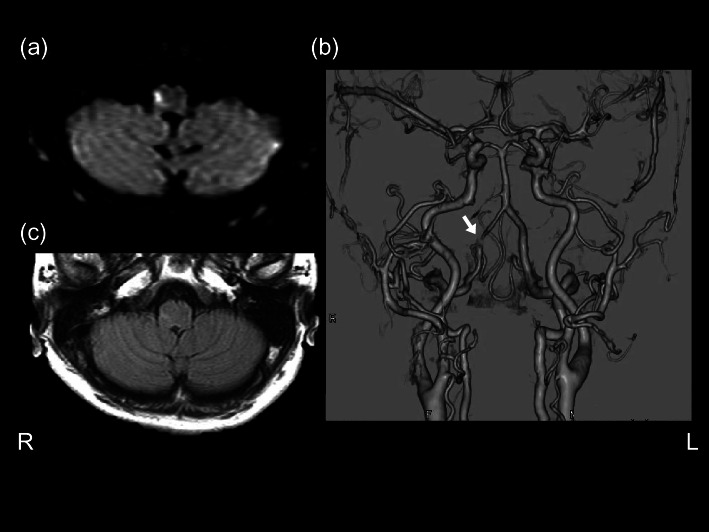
a Diffusion-weighted magnetic resonance images at the time of admission revealing a small high-intensity area in the midlateral portion of the right middle medulla. b Three-dimensional computed-tomography angiography (3D-CTA) performed 10 days after admission showing stenosis in the right vertebral artery. c Fluid-attenuated inversion recovery (FLAIR) MRI conducted 14 days after admission showing a faint high-intensity area almost exactly coinciding with the high-intensity area observed in diffusion-weighted images at admission.
